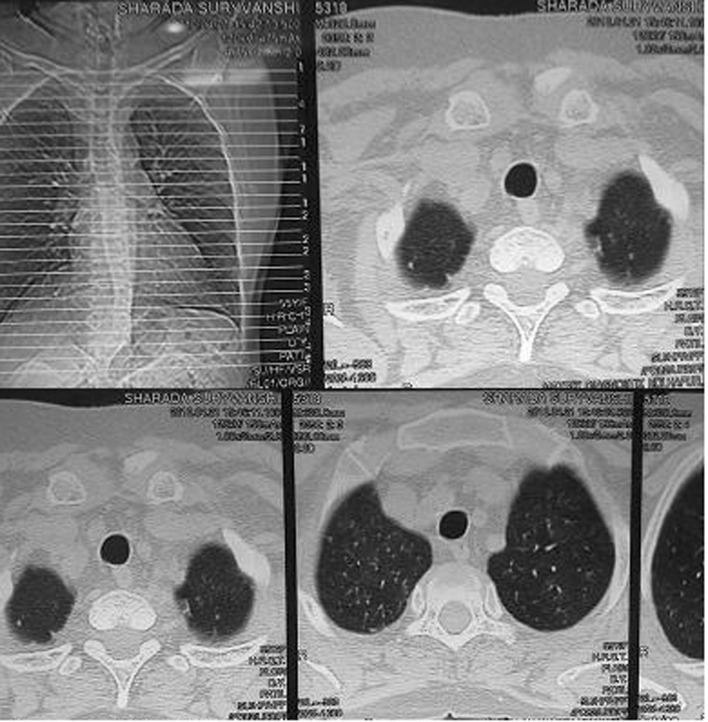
HRCT chest (22.04.2010) showing complete resolution of pulmonary shadows.
